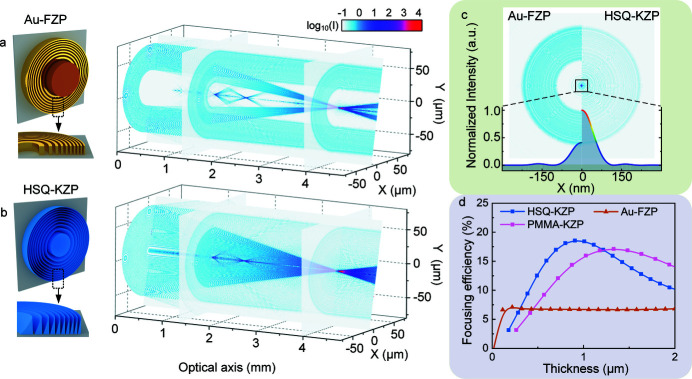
Comparisons of wavefield propagations between the HSQ kinoform zone plate (HSQ-KZP) and the metal zone plate (Au-FZP). The wavefield propagation is calculated using the beam propagation method combined with the quasi-discrete Hankel transform [BPM-QDHT (Tong *et al.*, 2022 ▸)]. The resolution of the Au-FZP (*a*) and the HSQ-KZP (*b*) is 100 nm with a diameter of 100 µm. The thickness of the Au-FZP and HSQ-KZP is 250 nm and 750 nm, respectively. The diameter of the beamstop for the FZP is 50 µm. (*c*) Comparison of point-spread functions of the focused light by both the Au-FZP and the HSQ-KZP, respectively, at the focal plane. (*d*) The calculated focusing efficiencies of the Au-FZP, HSQ-KZP and PMMA-KZP for soft X-rays (500 eV) using the BPM-QDHT approach.
